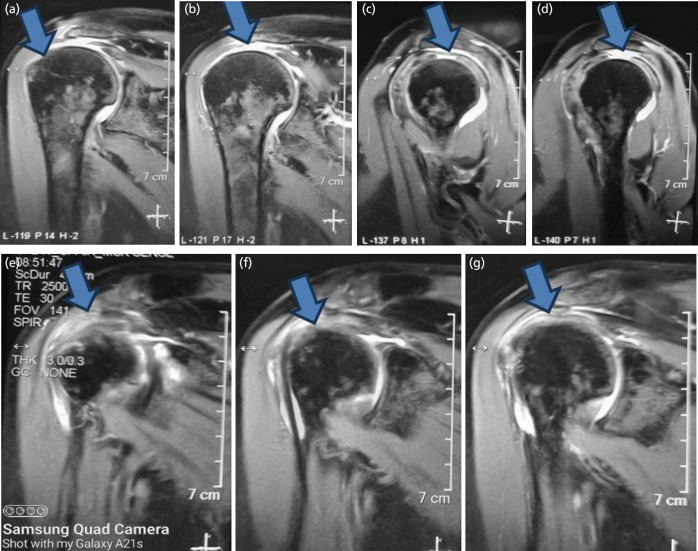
MRI images showing SAD (subacromial bursitis), tear supraspinatus and impingement of SST in diabetics. MRI Rt Shoulder in diabetic patients, (a to d) arrowhead showing complete tear of Supraspinatus in T1 weighted image, and SAD bursitis, (e to g) arrow SAD bursitis and impingement of Supraspinatus in T2 weighted image.
